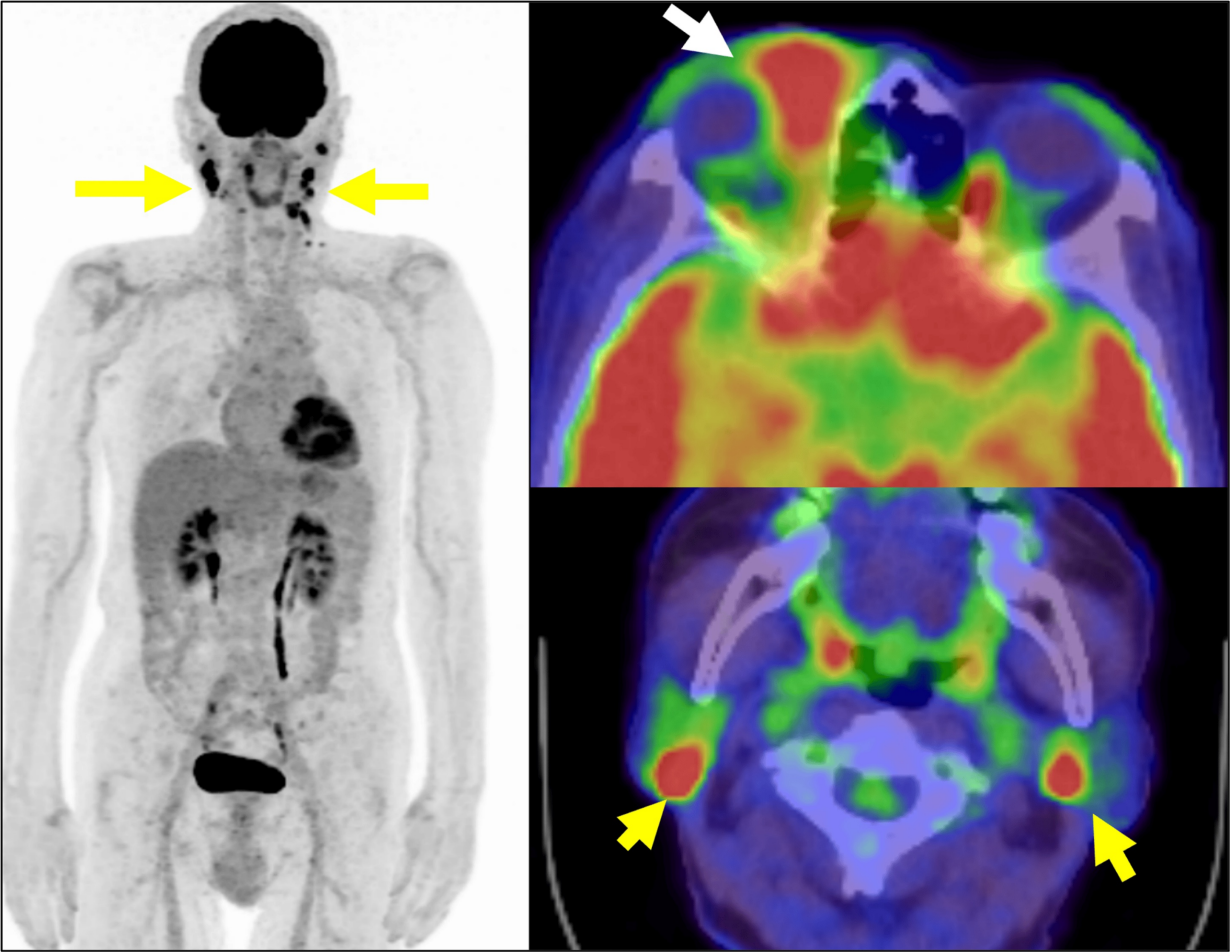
PET-CT findings of the right upper eyelid mass and bilateral cervical lymph nodes at initial diagnosis Positron emission tomography-computed tomography (PET-CT) imaging revealed intense fluorodeoxyglucose  (FDG) uptake in the right orbital mass (white arrow) and bilateral cervical lymph nodes (yellow arrows), with a maximum standardized uptake value (SUVmax) of 17.5. No other significant lymph node enlargement or abnormal FDG uptake was identified in other regions.
